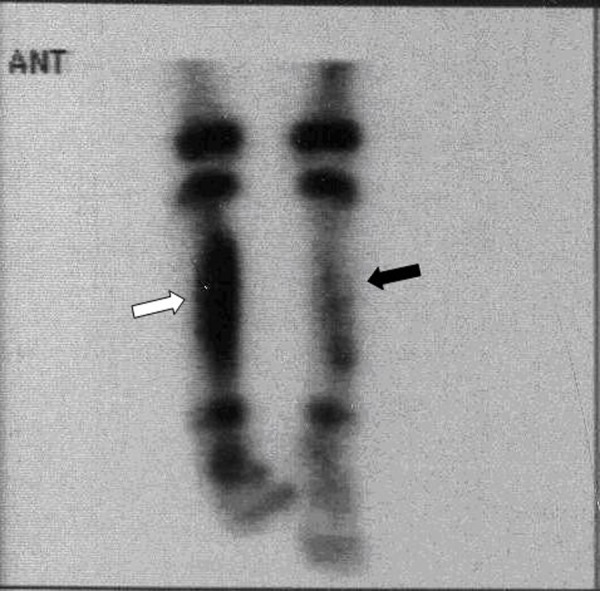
Bone scintigraphy three hours after i.v. injection Tc99m shows significantly increased uptake in right tibia (white arrow) and slightly increased uptake in the left tibial bone (black arrow).
